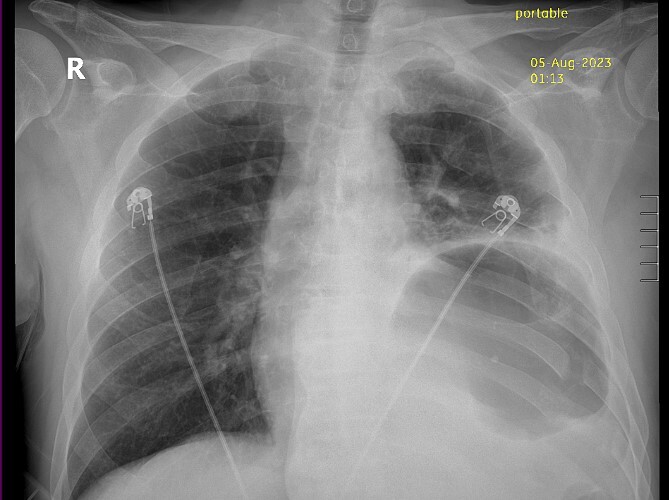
Chest X-ray showing gastric body in the left hemithorax.
